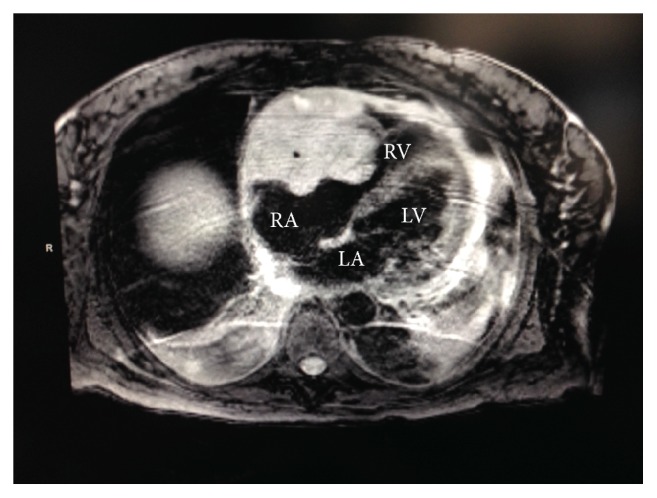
Cardiac MRI image showing a large lobulated heterogenous mass in the right ventricle which limited the opening of the tricuspid valve.
